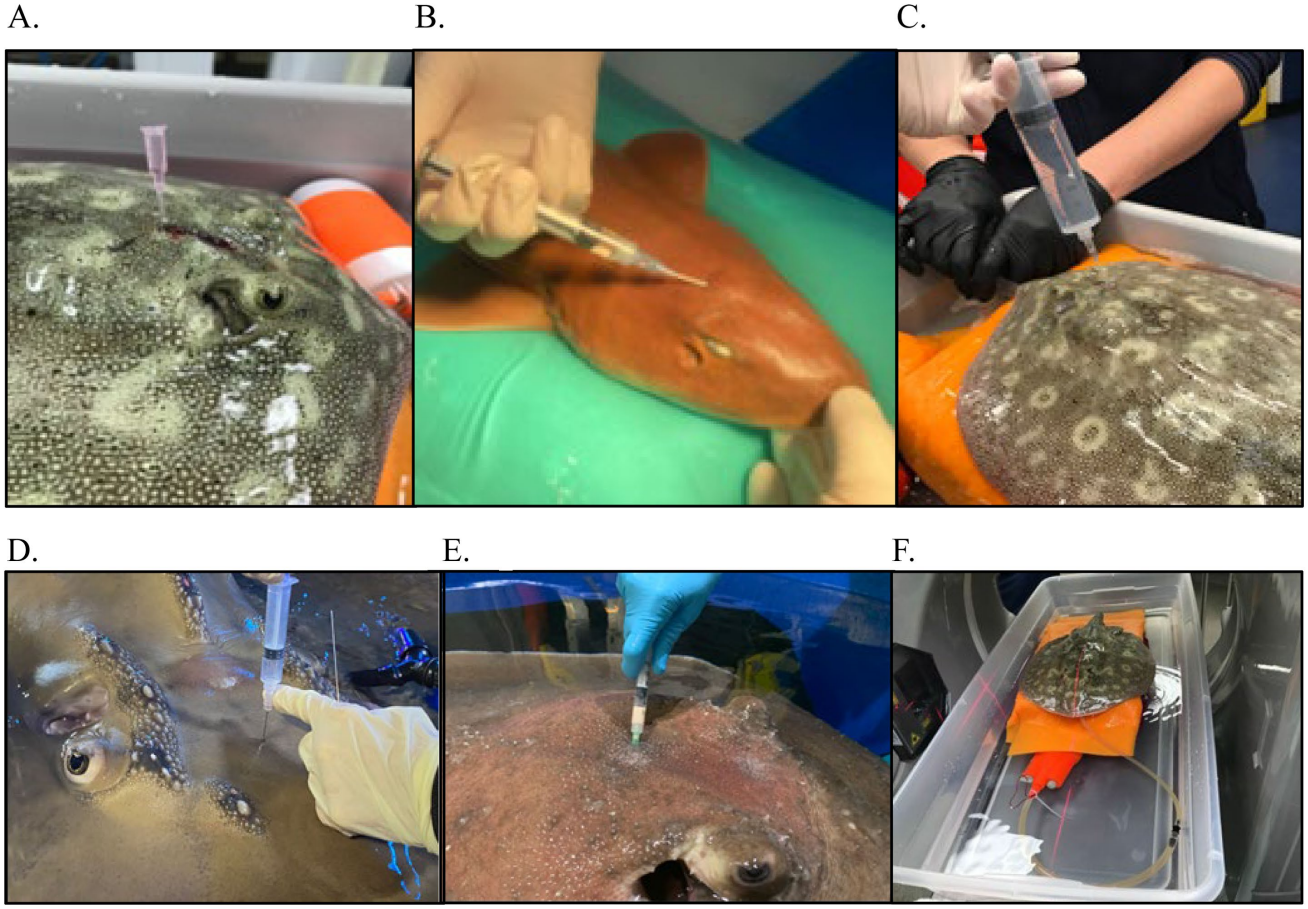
Examples of various diagnostics for endolymphatic disease in elasmobranchs. (A) Marking of affected area for sample collection and treatment administration in a yellow ray (*Urobatis jamaicensis*); (B) Flushing of affected region as part of debridement in a white-spotted bamboo shark (*Chiloscyllium plagiosum*); (C) Flushing the affected region as part of debridement in a yellow ray (*Urobatis jamaicensis*); (D) Abscess aspiration in a porcupine ray (*Urogymnus asperrimus*); (E) Cerebrospinal fluid (CSF) collection in a bowmouth guitarfish (*Rhina ancylostoma*); (F) Cone-beam computed tomography of an anesthetized yellow ray (*Urobatis jamaicensis*), ventilated while raised out of water to maximize image quality.
